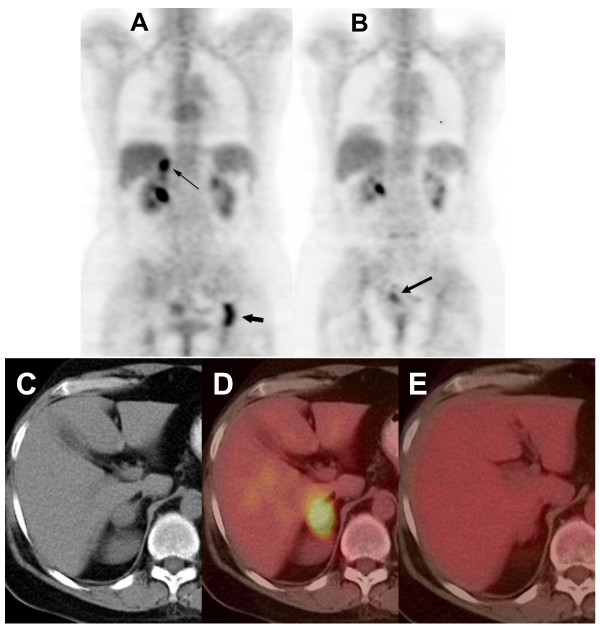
59 yo postmenopausal female with ER/PR positive, ERBB2 negative breast cancer with biopsy confirmed metastasis to the left ischium and right adrenal gland. A) Baseline PET MIP image demonstrates metastatic disease of the right adrenal gland (small arrow) and the left ischium (large arrow). B) PET MIP image four months after baseline shows the FDG activity in the right adrenal and left ischium has resolved indicating response to therapy. However, a primary uterine tumor, which was barely detectable in the baseline study, grew during the same time frame (arrow). C) Baseline PET/CT (left panel): The non-contrast CT shows an enlarged right adrenal gland. D) Baseline PET/CT (right panel): The fused PET/CT demonstrates increased FDG activity in the enlarged right adrenal gland. E) Follow-up PET/CT: The fused PET/CT four months after baseline shows a decrease in FDG activity of the right adrenal gland. Note the corresponding decrease in size also.
